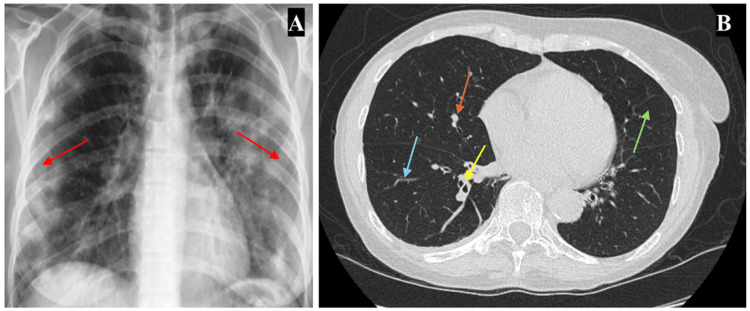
(A) Chest X-ray shows peripheral airspace opacities sparing the perihilar region (reverse batwing sign), as indicated by red arrows. (B) Chest CT shows small pulmonary nodules (orange arrow), vascular walls with mural enhancement (light blue arrow), persistent lower lobe bronchial wall thickening (yellow arrow), and mild emphysema (green arrow).
